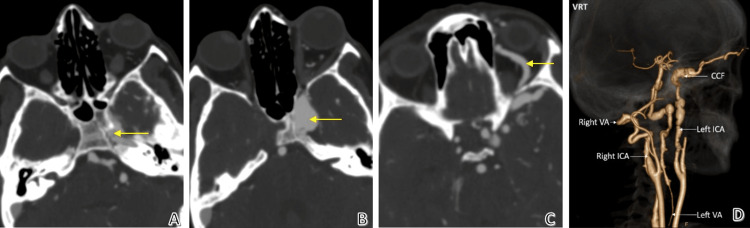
CT angiography findings Post-contrast CT images show a linear filling defect in the left cavernous ICA (yellow arrow) due to dissection (A), outward bulging of the left cavernous sinus (yellow arrow) due to caroticocavernous fistula (B), a prominent left superior ophthalmic vein (yellow arrow) (C), and a VRT image depicting narrowing and ectasia at various levels in both the carotid and vertebral arteries (D).
